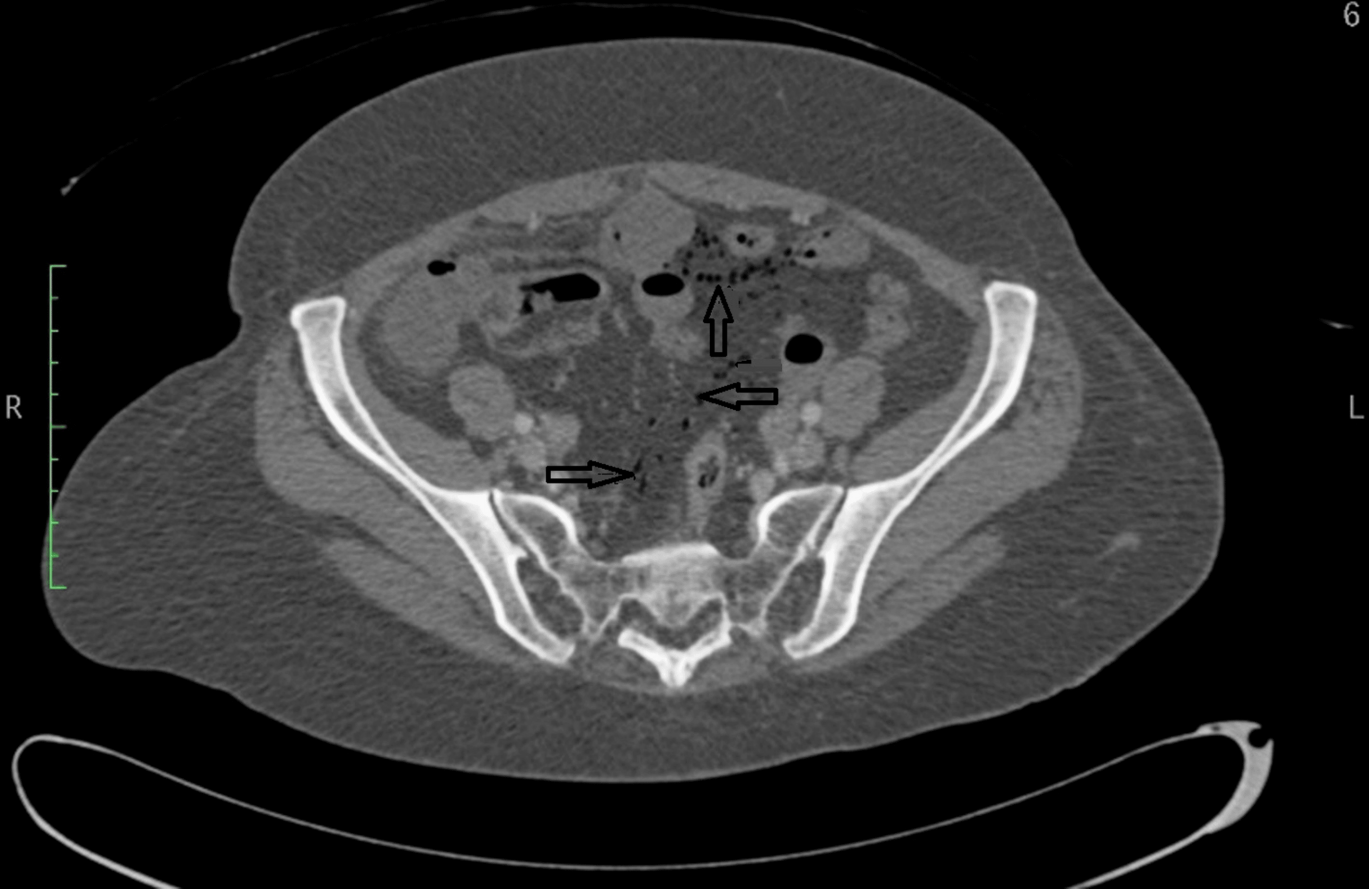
Cross-sectional CT scan of the abdomen and pelvis showing diffuse pneumatosis intestinalis (black arrows)
